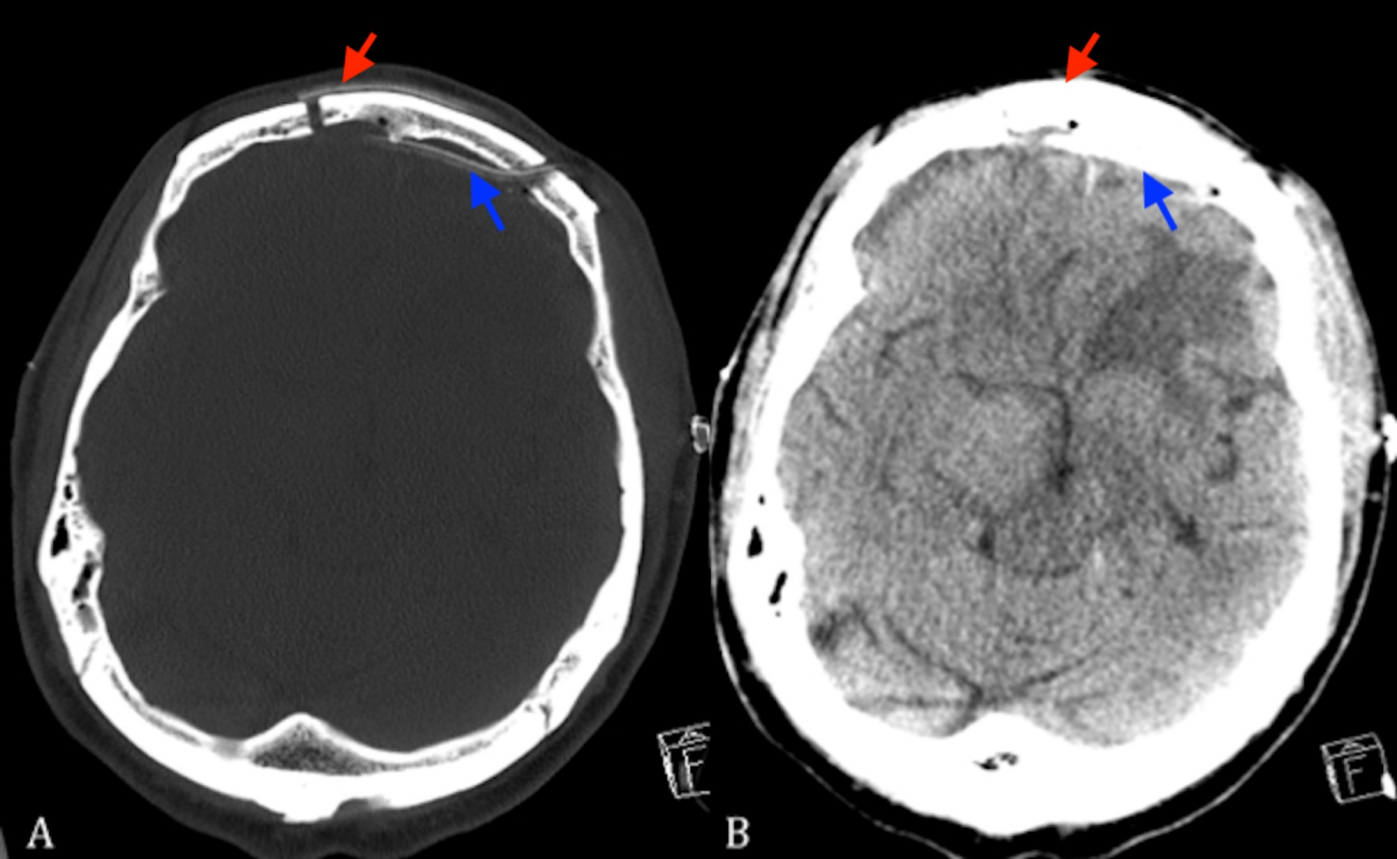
(A) Bone windowed axial non-contrast head CT. (B) Brain windowed axial non-contrasted head CT scan showing placement of subgaleal (red arrow) and epidural catheters (blue arrow). CT: Computed tomography
